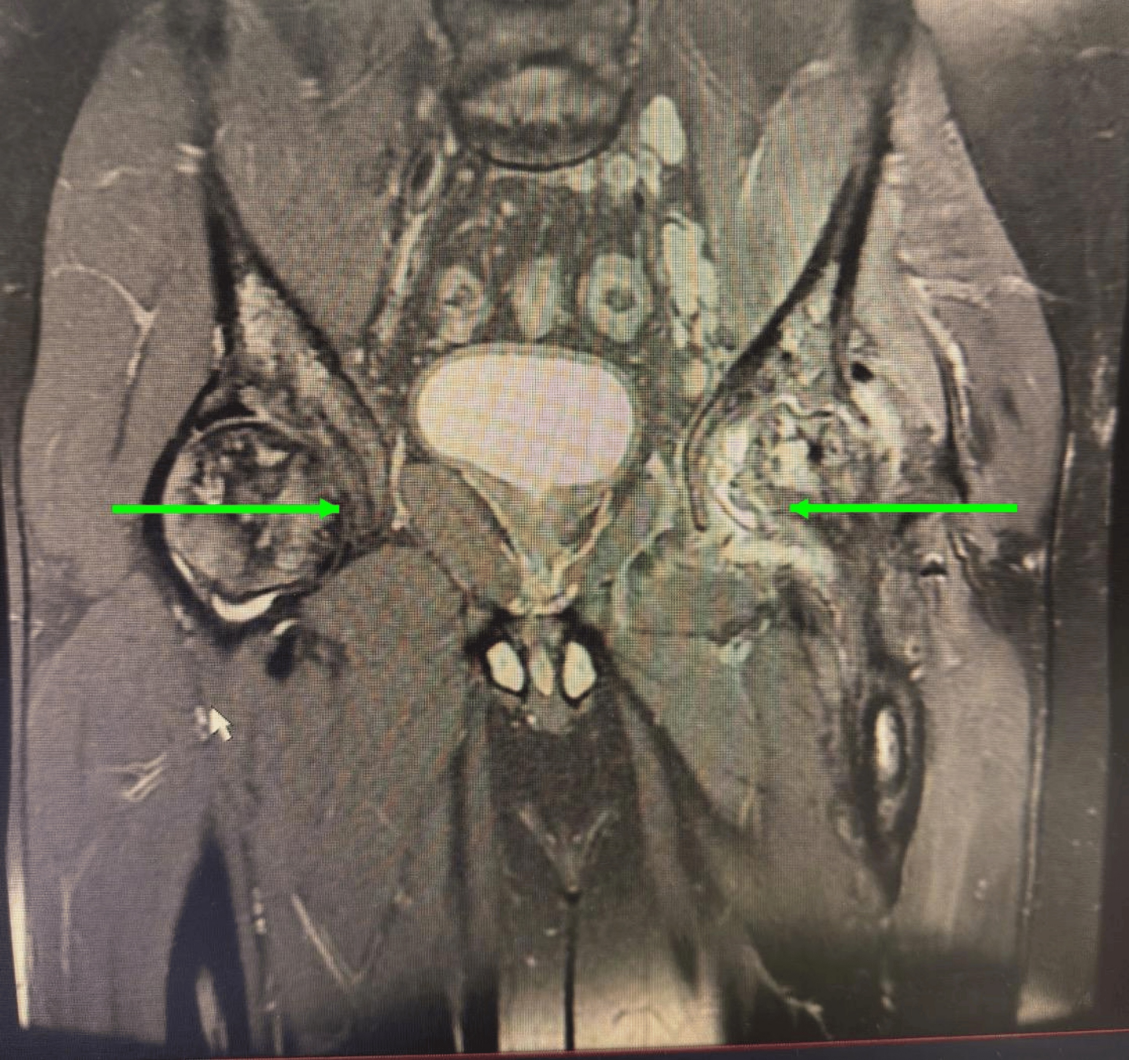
Contrast-enhanced magnetic resonance imaging showing bilateral femoral head osteonecrosis, with additional findings of left proximal femoral osteomyelitis and septic arthritis of the left hip associated with intraosseous and peri-osseous communicating abscess formation. The right green arrow shows left proximal femoral osteomyelitis and septic arthritis with associated intraosseous and peri-osseous communicating abscess formation, and the left green arrow shows right femoral head osteonecrosis.
